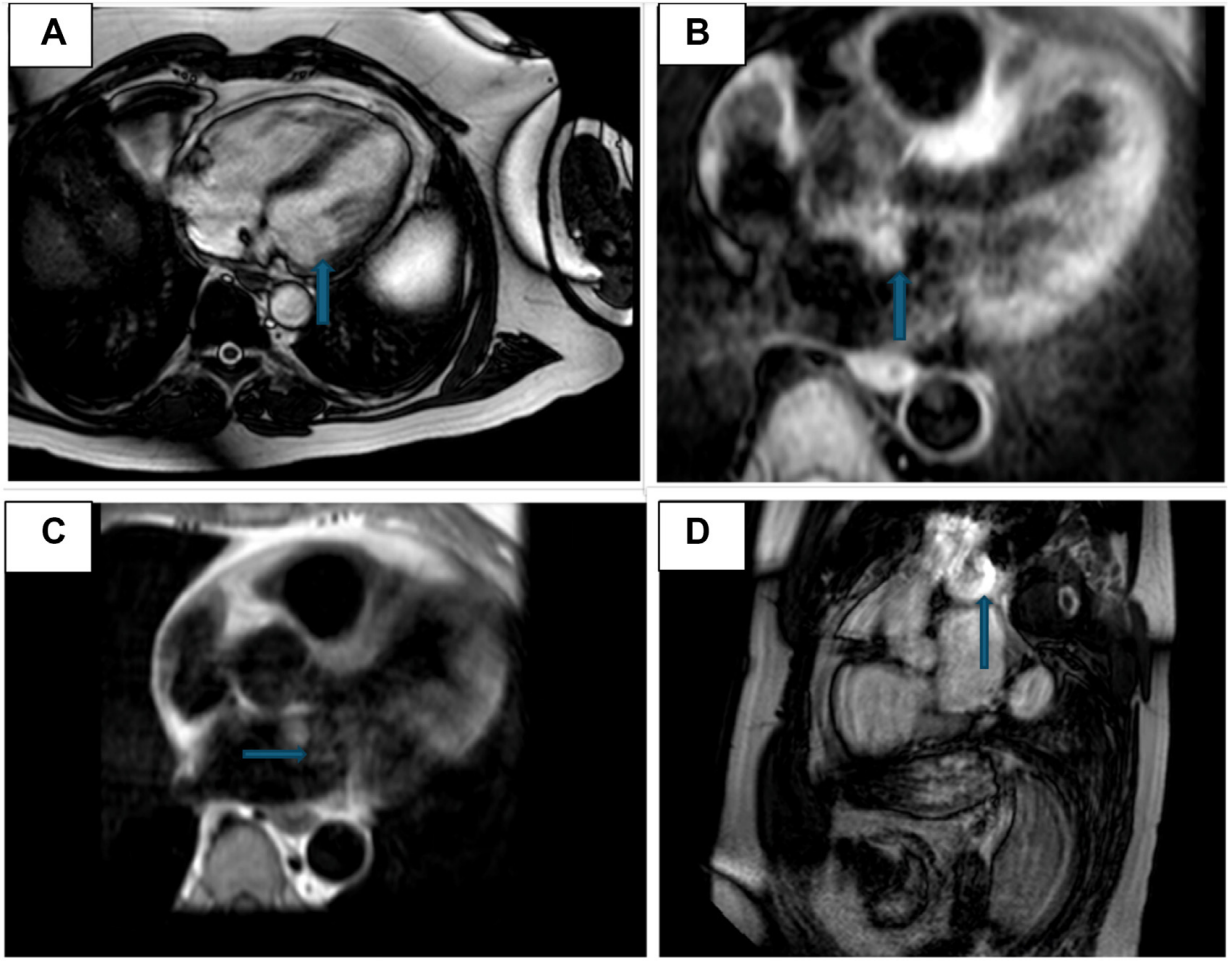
Cardiac Magnetic Resonance Imaging Cardiac magnetic resonance imaging (MRI) shows a 15 × 14 mm mass in the left atrium along the anteromedial wall (A), indicated by an arrow. The lesion appears isointense to skeletal muscle on T1-weighted images (B, C) and hyperintense on the T2-weighted sequence (D), as highlighted by arrows. Its location adjacent to the aortic root and imaging characteristics are highly suggestive of a myxoma.
